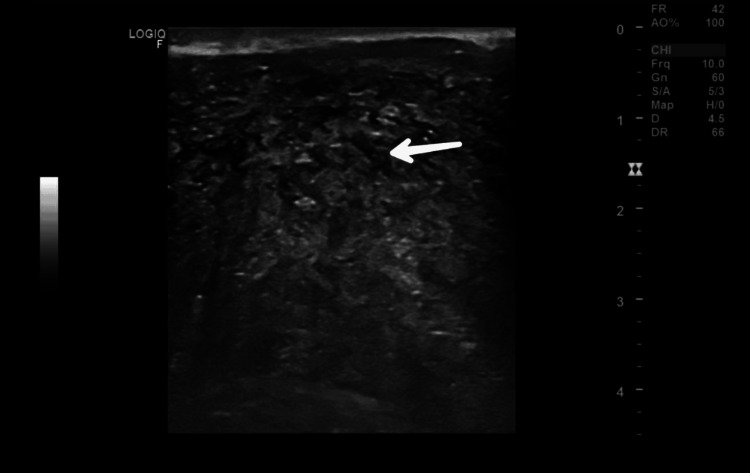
Breast ultrasound image of case 2 Ultrasound of the breast shows a large, relatively well-defined mass lesion involving all quadrants (arrow) (BI-RADS IV). BI-RADS: Breast Imaging Reporting and Data System
